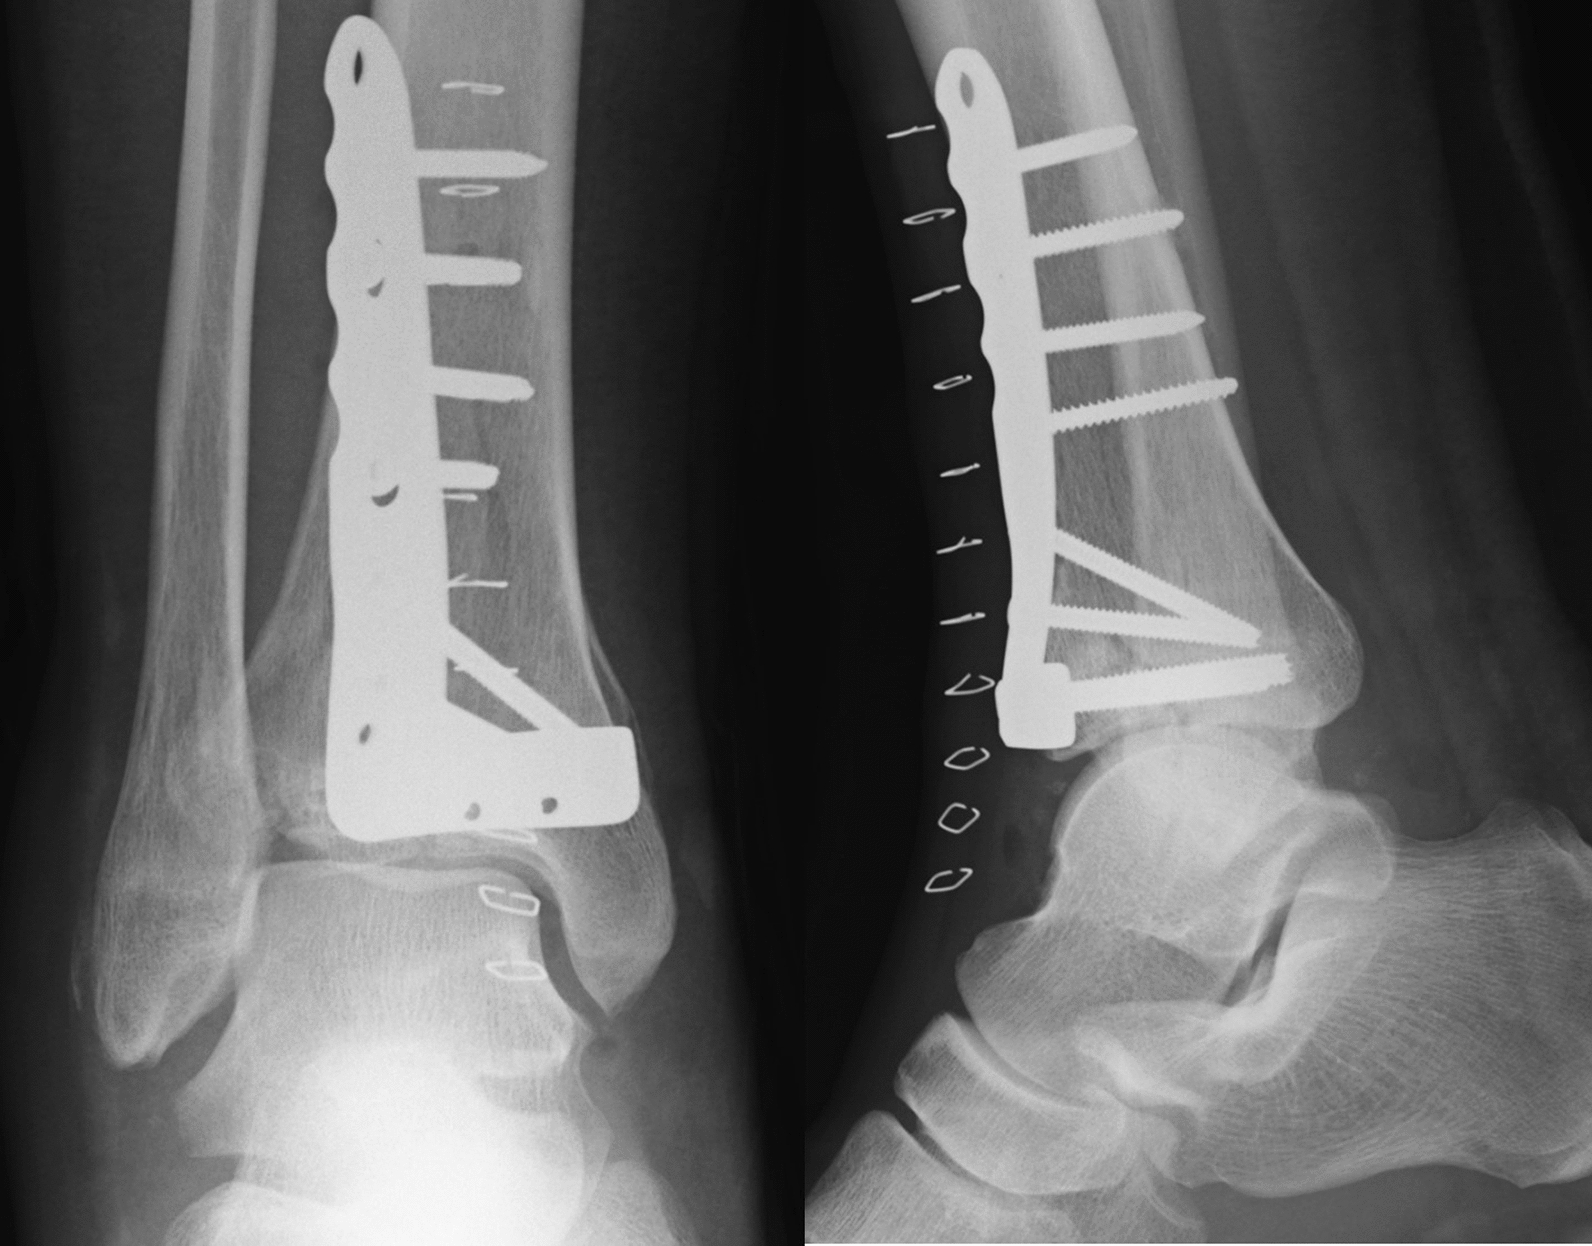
Plain radiographs of right ankle after the fixation; (right) anteroposterior view and (left) lateral view
